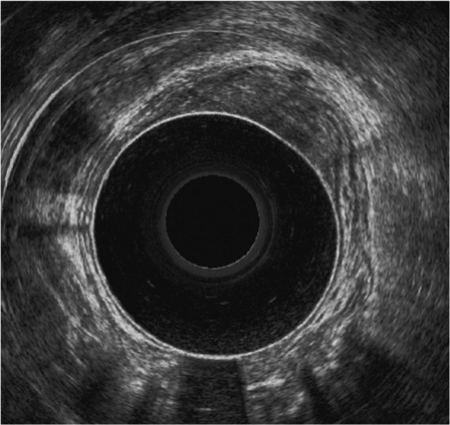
Rectal endosonography with a water balloon. A tumor limited to the mucosa, not invading the submucosa and muscularis propria.
